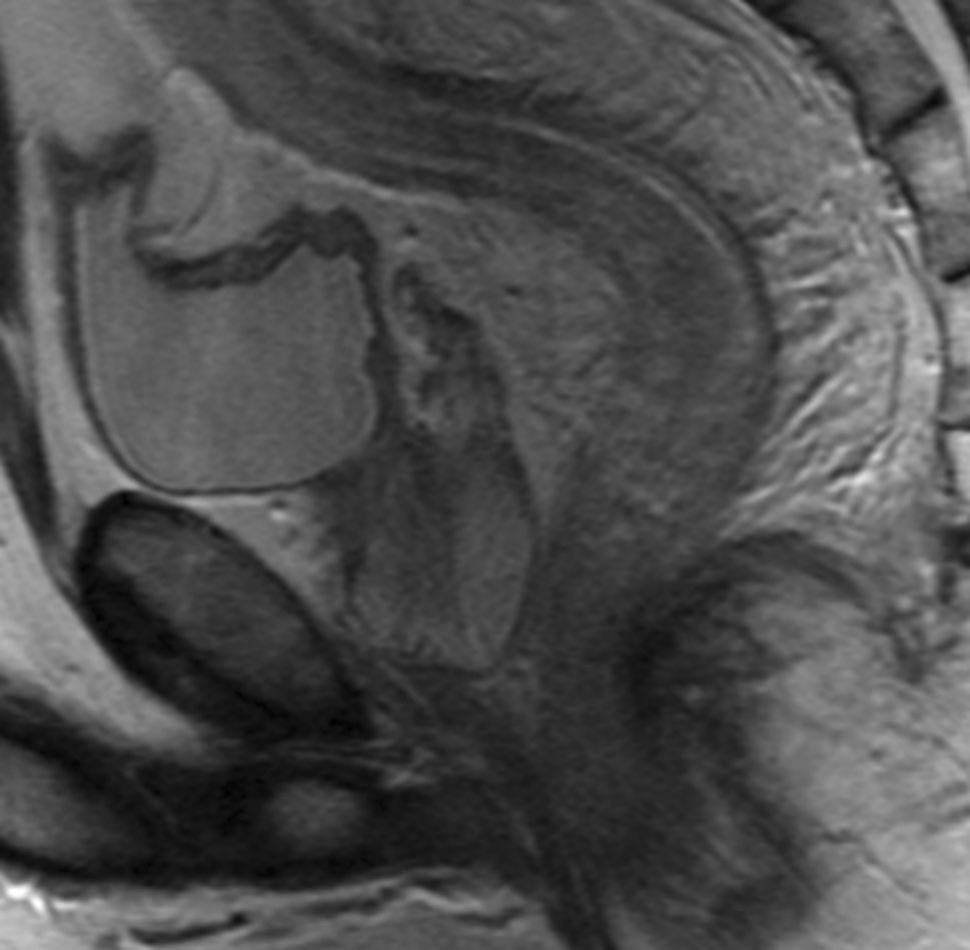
A 43-year-old male with Crohn’s disease and ulcerative proctitis at endoscopy. Sagittal T2-weighted image shows the increased perimural vascularity perpendicular to the rectum (‘comb sign’) in addition to the wall thickening of the entire rectum
